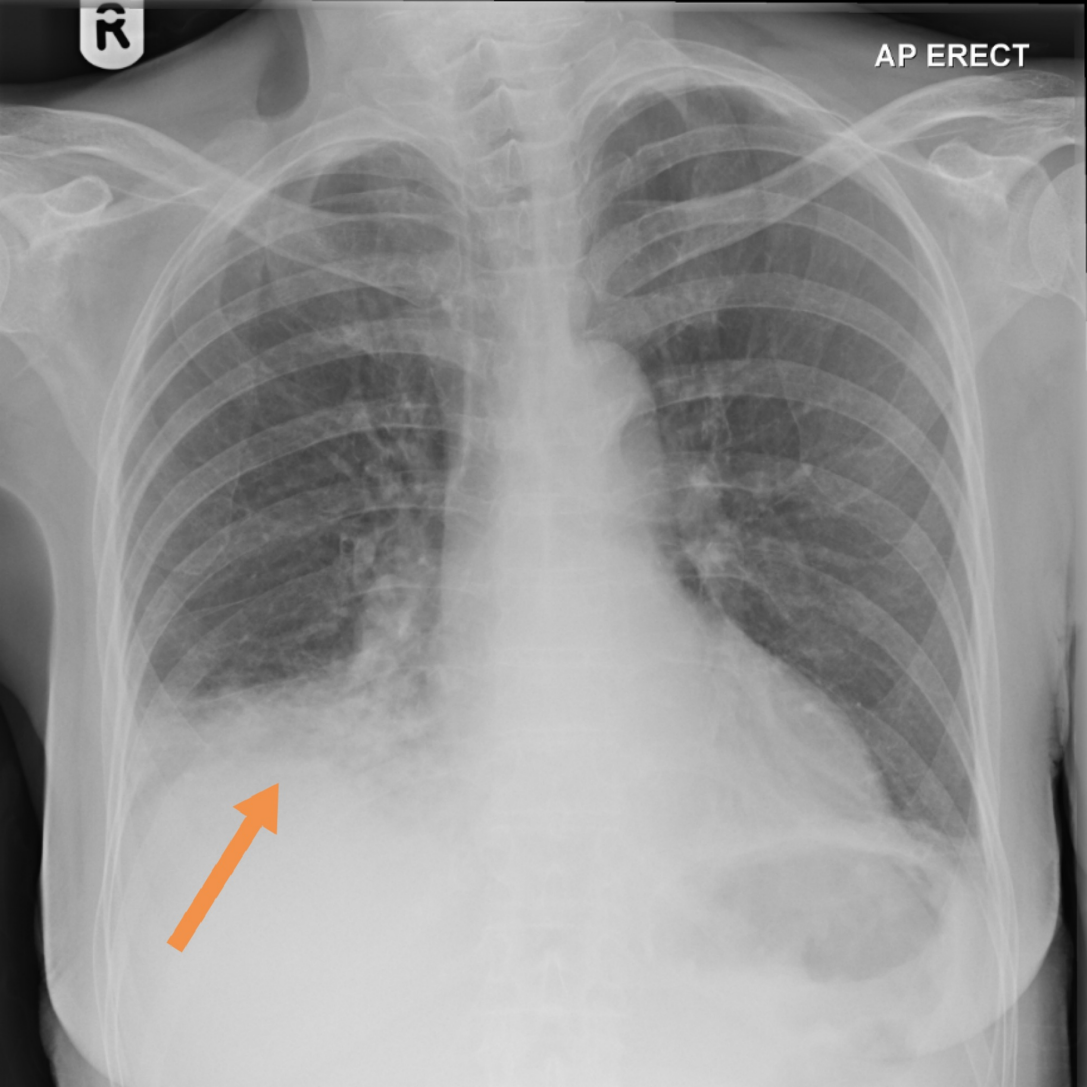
Chest x-ray on admission showing right lower lobe consolidation (see arrow)
